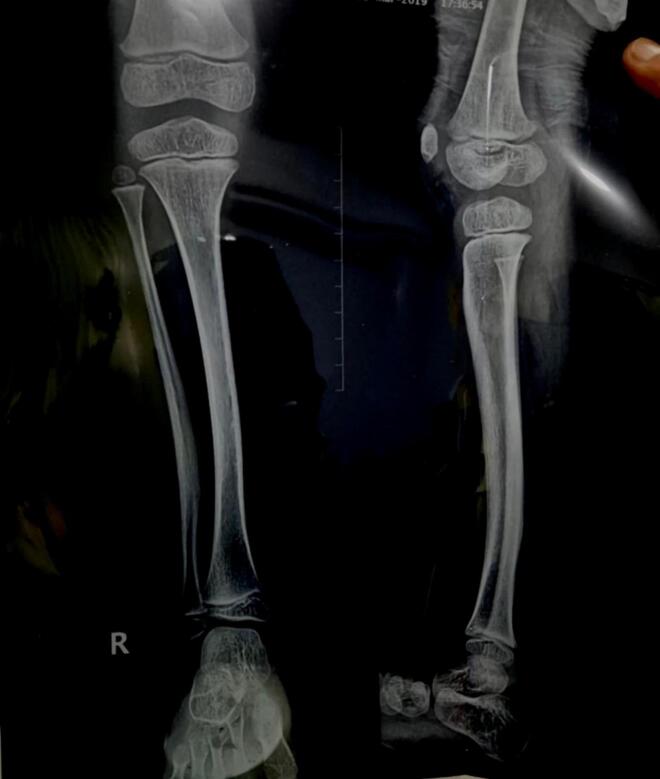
X-ray of Right leg (anteroposterior and lateral views) showing anterolateral bowing of the right tibial diaphysis. The X-ray dates back to when the patient was 3 years old.
